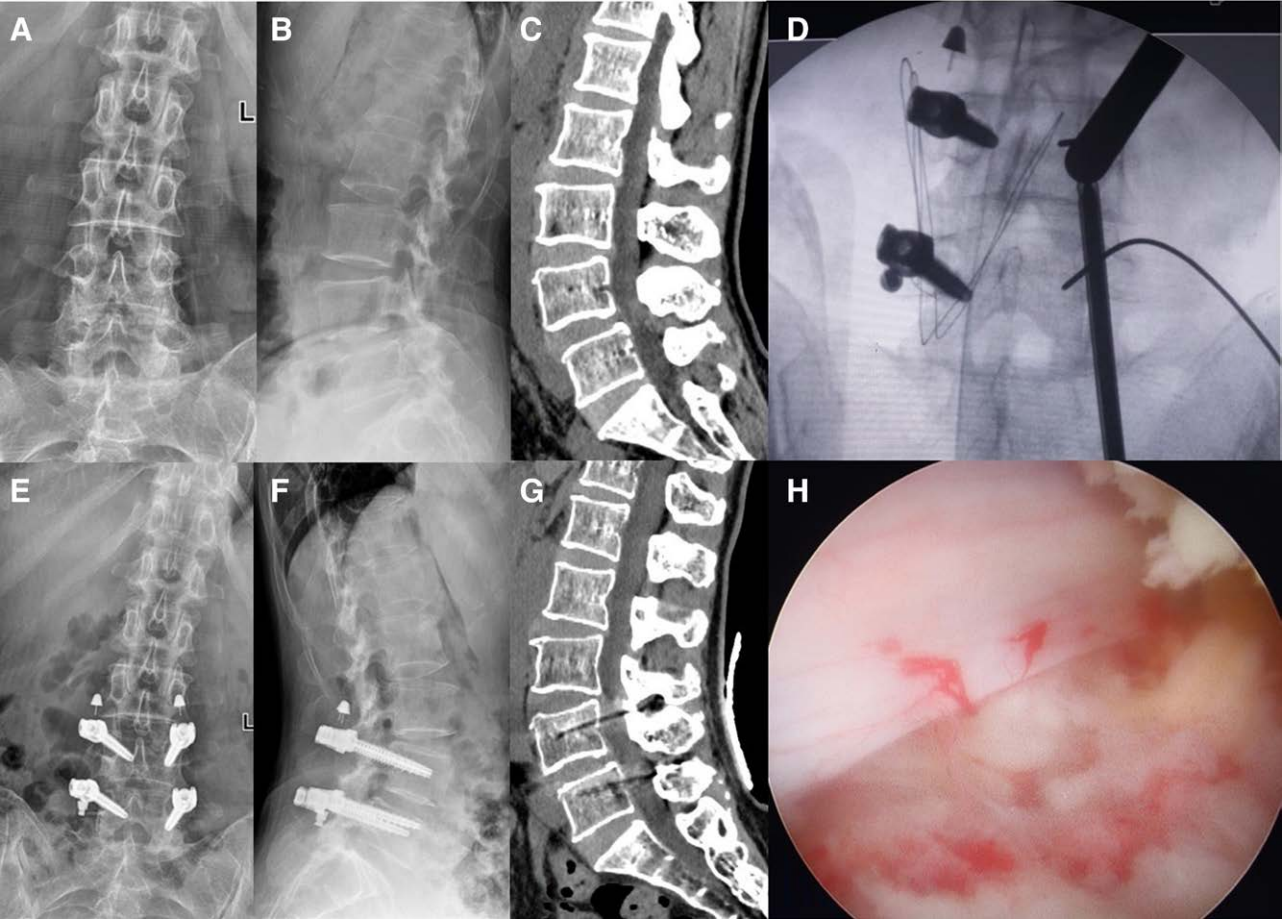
(A–C) Preoperative lumbar spine anteroposterior and lateral X-rays and lumbar spine CT sagittal images showing mild anterior slippage of the L4 vertebral body. (E–G) Postoperative follow-up lumbar spine anteroposterior and lateral X-rays and lumbar spine CT sagittal images showing good placement of screws and PEEK rod. (D) Left pedicle screw and mock rod inserted during surgery, right side secured with UBE working sleeve. (H) Nerve root photo taken after satisfactory decompression. CT = computerized tomography.
